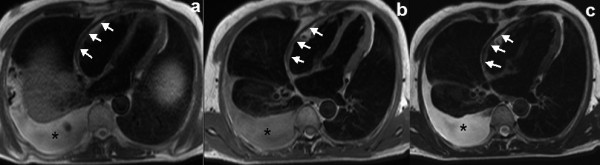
Diffuse thickened pericardium. HASTE (A), T1- (B) and T2-weighted (C) TSE images showed diffuse pericardial thickening (white arrows) which is most pronounced over the RV and RA and moderate right-sided pleural effusion (*).
